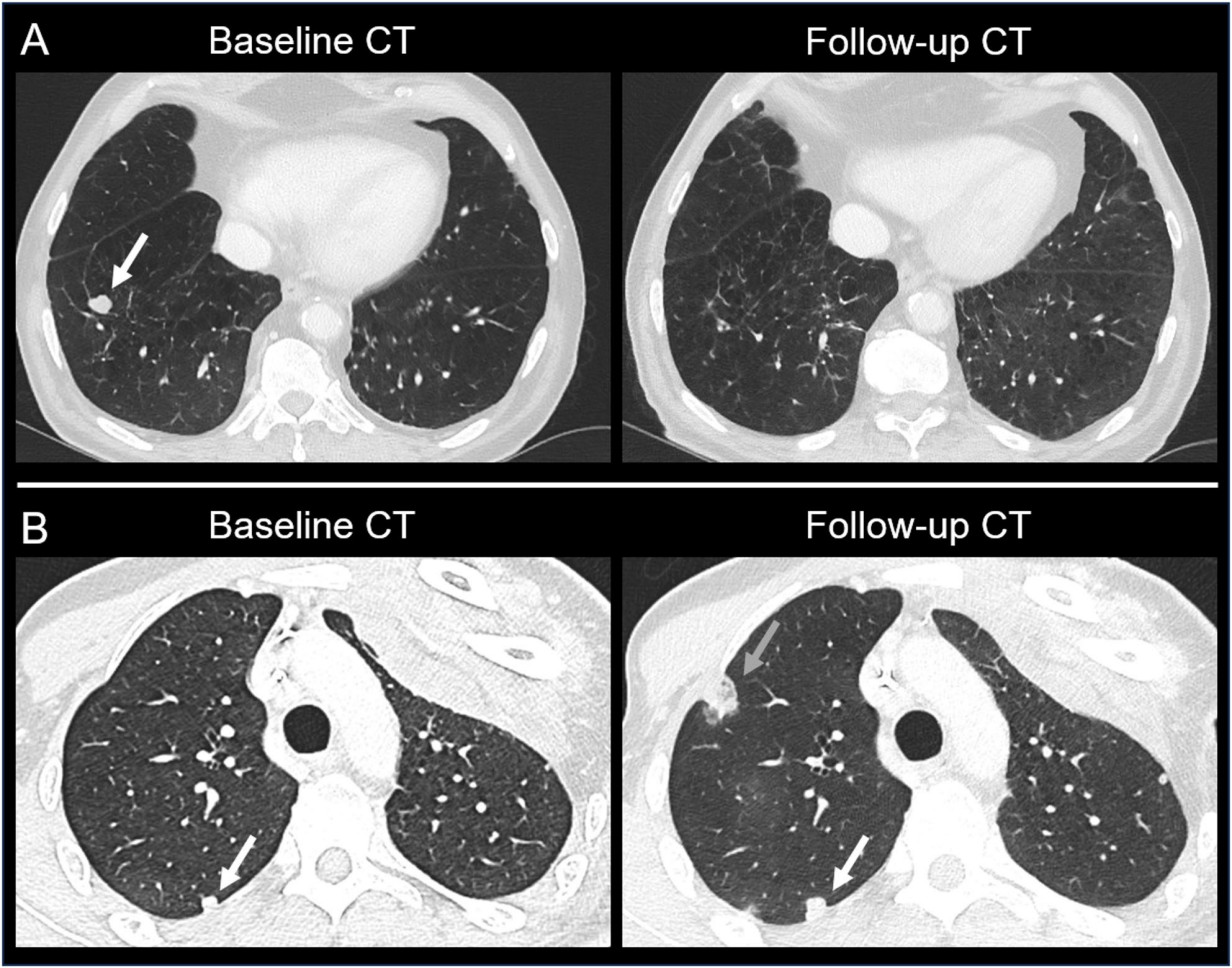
CT imaging of the lung for sarcoma patients with regressive pulmonary metastasis (A) and progressive pulmonary metastasis (B). Baseline CT of the patient shown on top revealed a singular metastasis in the right upper lobe (white arrow) which showed near-complete remission in follow-up CT after 95 days. Baseline CT of the patient shown at bottom revealed multiple metastases (*n* > 5). The white arrow points at an exemplary metastasis at the right upper lobe which had a unidimensional tumor growth of 27% in follow-up CT after 29 days. The grey arrow indicates an additional metastasis which was not present in baseline CT imaging. Overall survival was 267 (A) and 68 days (B), progression-free survival was 197 (A) and 29 days (B)
